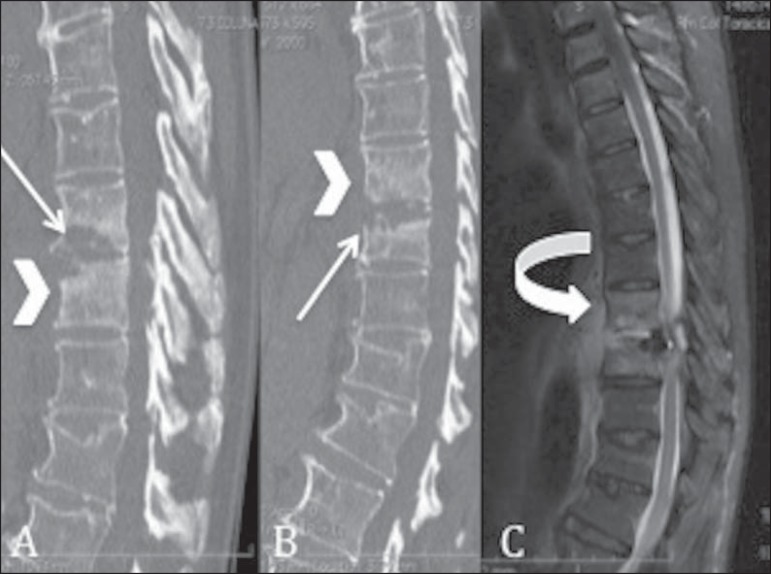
Computed tomography with sagittal reconstructions
